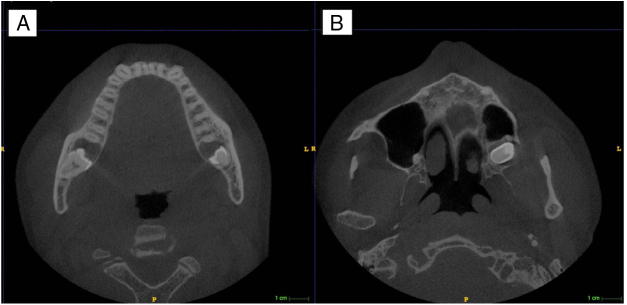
CBCT axial scans (A and B) showing bucco-lingual expansion and the cortical thinning in the mandible involving the third molars (A) and the upper left third molar (B).
